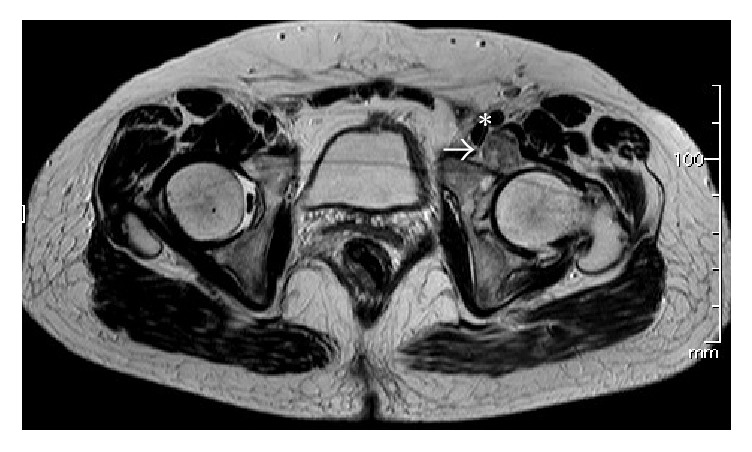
T1-weighted axial image of extra-articular loose bodies (arrow) adjacent to the iliopsoas muscle and the femoral vessels (asterisk).
